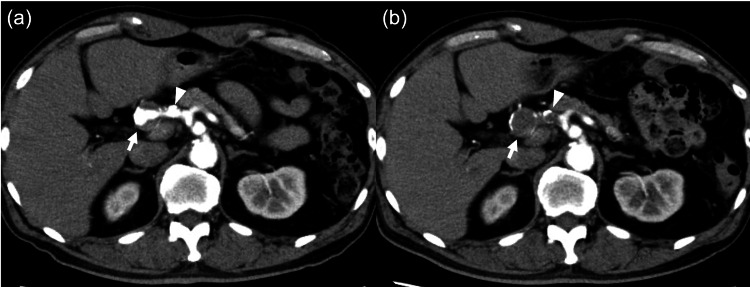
Follow-up contrast-enhanced CT at 5 years and 5.5 years (a) Arterial-phase contrast-enhanced CT image obtained 5 years after the initial diagnosis. The overall aneurysm size remains largely unchanged; however, increased intraluminal thrombosis is observed compared with baseline (arrows). The dissection-related change in the proximal segment (arrowhead) appears largely unchanged compared with the initial examination. (b) Arterial-phase contrast-enhanced CT image obtained 6 months later (5.5 years after the initial diagnosis). Complete thrombosis of the aneurysmal lumen is demonstrated (arrows). The previously identified dissection-related proximal segment is also thrombosed on this examination (arrowhead). Hepatic parenchymal enhancement is preserved, suggesting adequate collateral arterial perfusion.
